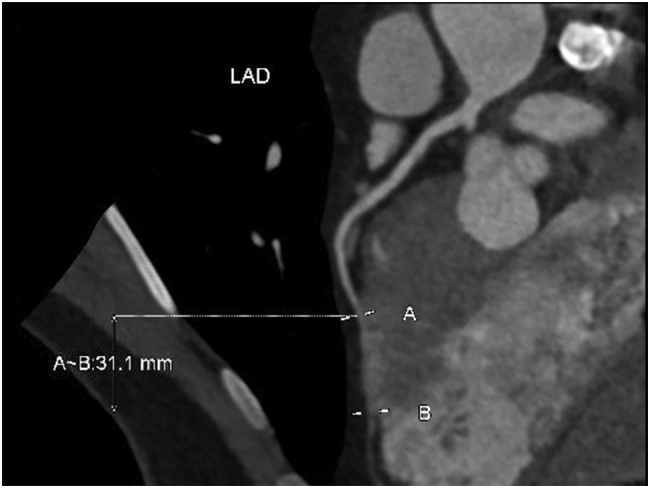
CTA showing 3.1 mm LAD myocardial bridge.
